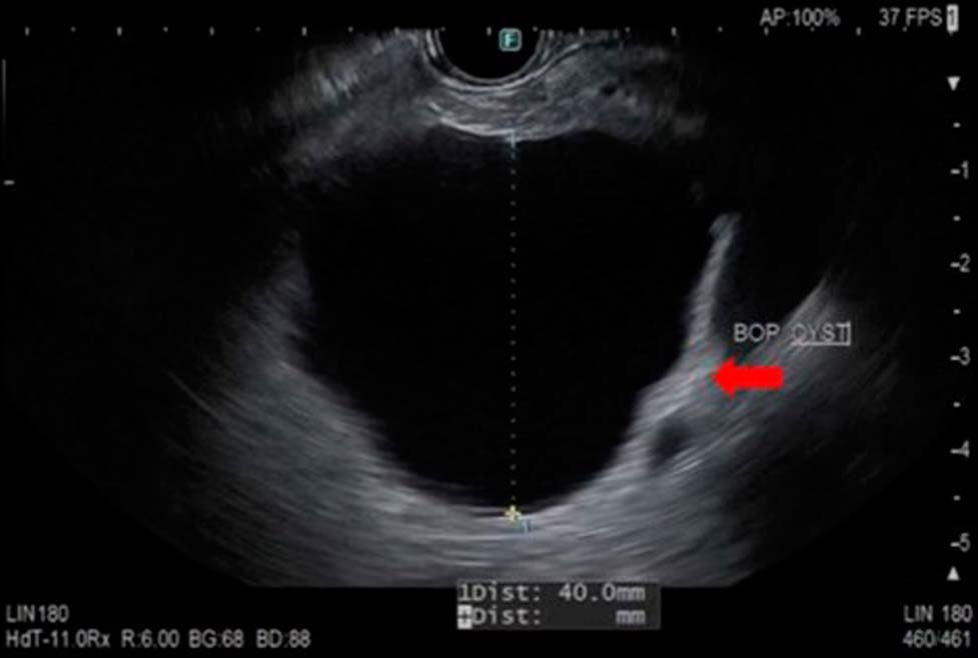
Endoscopic ultrasound of the largest cyst in the distal pancreatic body. The cyst was nonseptated and anechoic with subtle ductal communication. No high-risk features such as solid component or mural nodule were seen.
